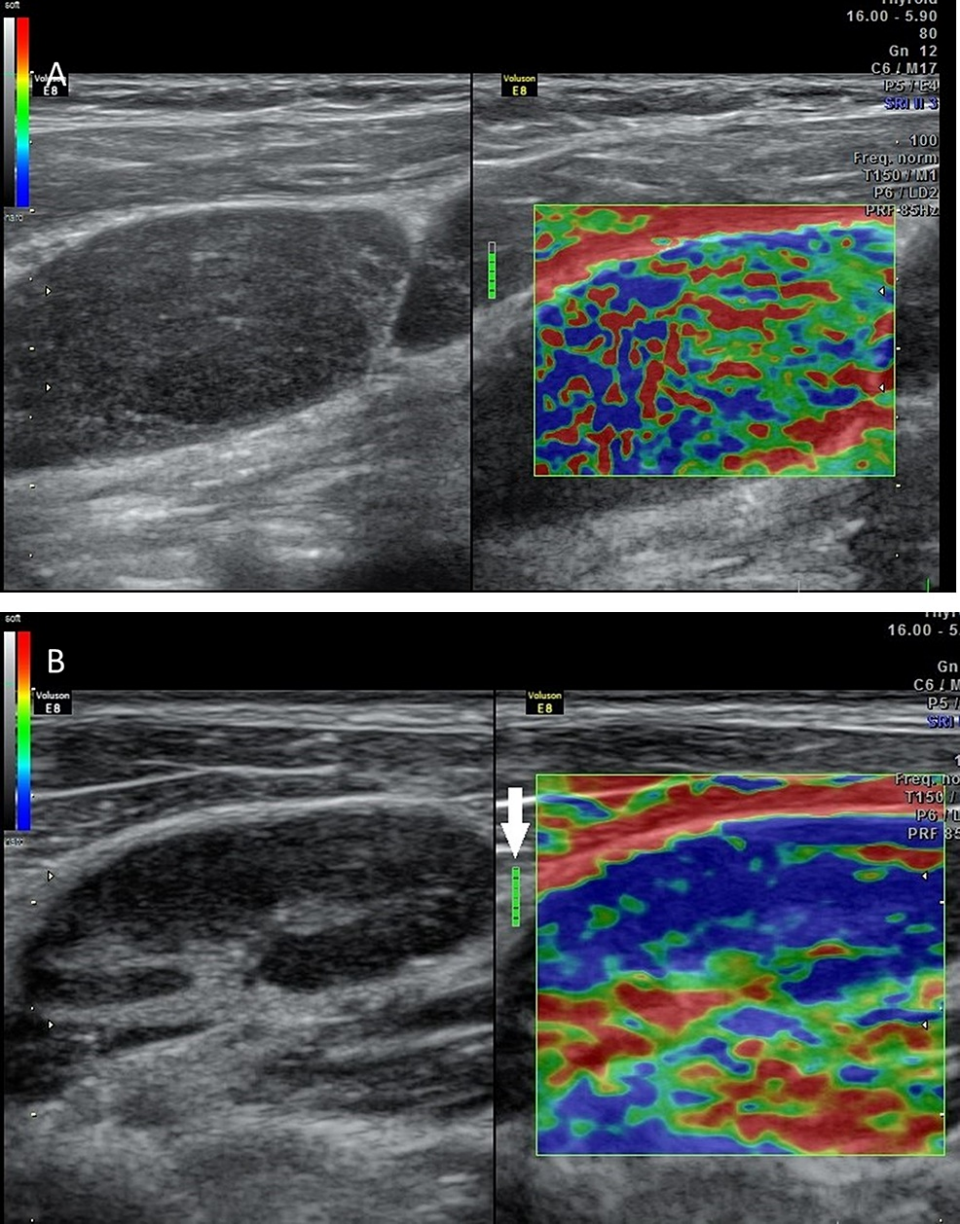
Sonoelastography images of cervical lymph nodes. (A) Ultrasound elastogram image showing pattern I suggestive of benign etiology; (B) ultrasound elastogram showing pattern IV suggestive of malignant etiology. The green colour in the feedback indicator (white arrow) indicates adequate compression.
